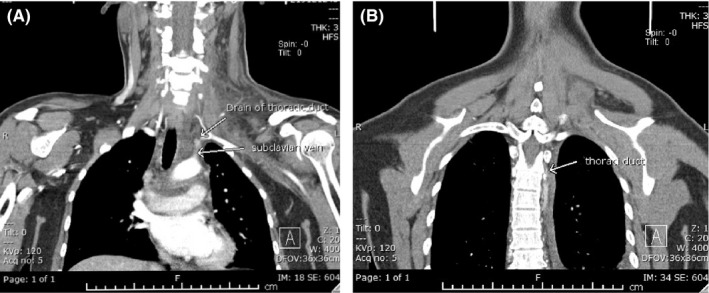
A and B, CT scan showing left neck subcutaneous edema, the track of thoracic duct
